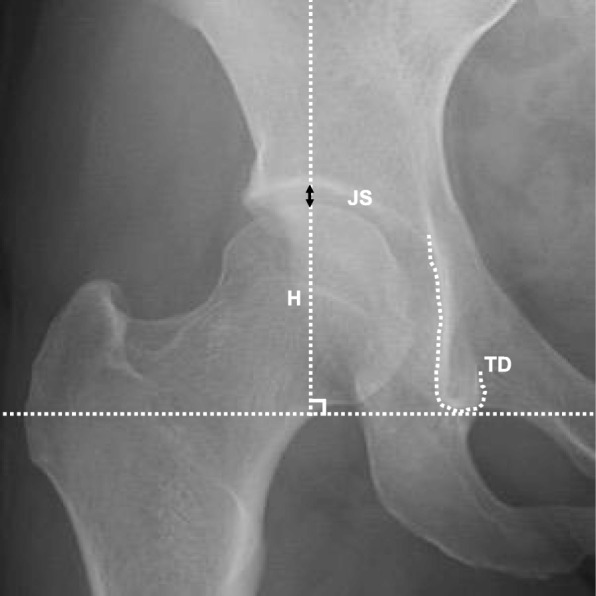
Method of joint space measurement by radiographs. Measurement of the joint space (JS) in the anteroposterior view of the radiographs. JS measured between the femur and acetabulum on the perpendicular line passing the femoral head (H) from the connecting line of both teardrops (TD)
